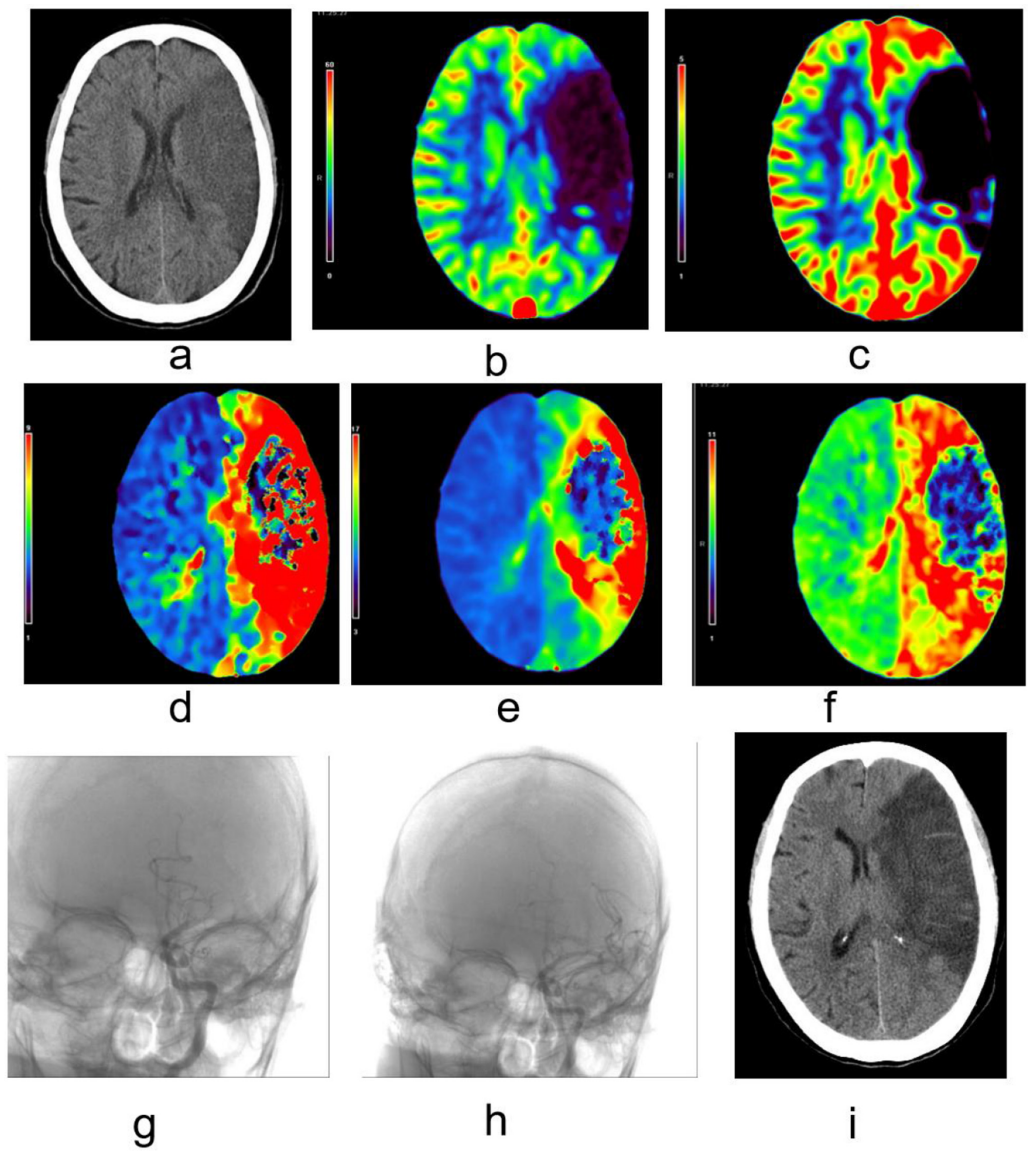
Typical case images; (a) Low-density infarct in the left frontal, temporal, and parietal lobes, as well as the left basal ganglia and centrum semiovale; (b) Reduced cerebral blood flow (CBF) in the distribution of the left middle cerebral artery; (c) Reduced cerebral blood volume (CBV) in the distribution of the left middle cerebral artery; (d) Prolonged mean transit time (MTT) in the distribution of the left middle cerebral artery; (e) Prolonged time to peak (TTP) in the distribution of the left middle cerebral artery; (f) Prolonged delay in the distribution of the left middle cerebral artery, indicating perfusion deficits; (g) Pre-thrombectomy DSA confirming occlusion in the M1 segment of the left middle cerebral artery; (h) Post-thrombectomy DSA showing mTICI level 3 reperfusion, indicating successful restoration of blood flow in the left middle cerebral artery; (i) Post-surgery non-contrast CT showing a decrease in infarct density with more distinct margins, indicating improvement in tissue perfusion and reduction in ischemic damage.
